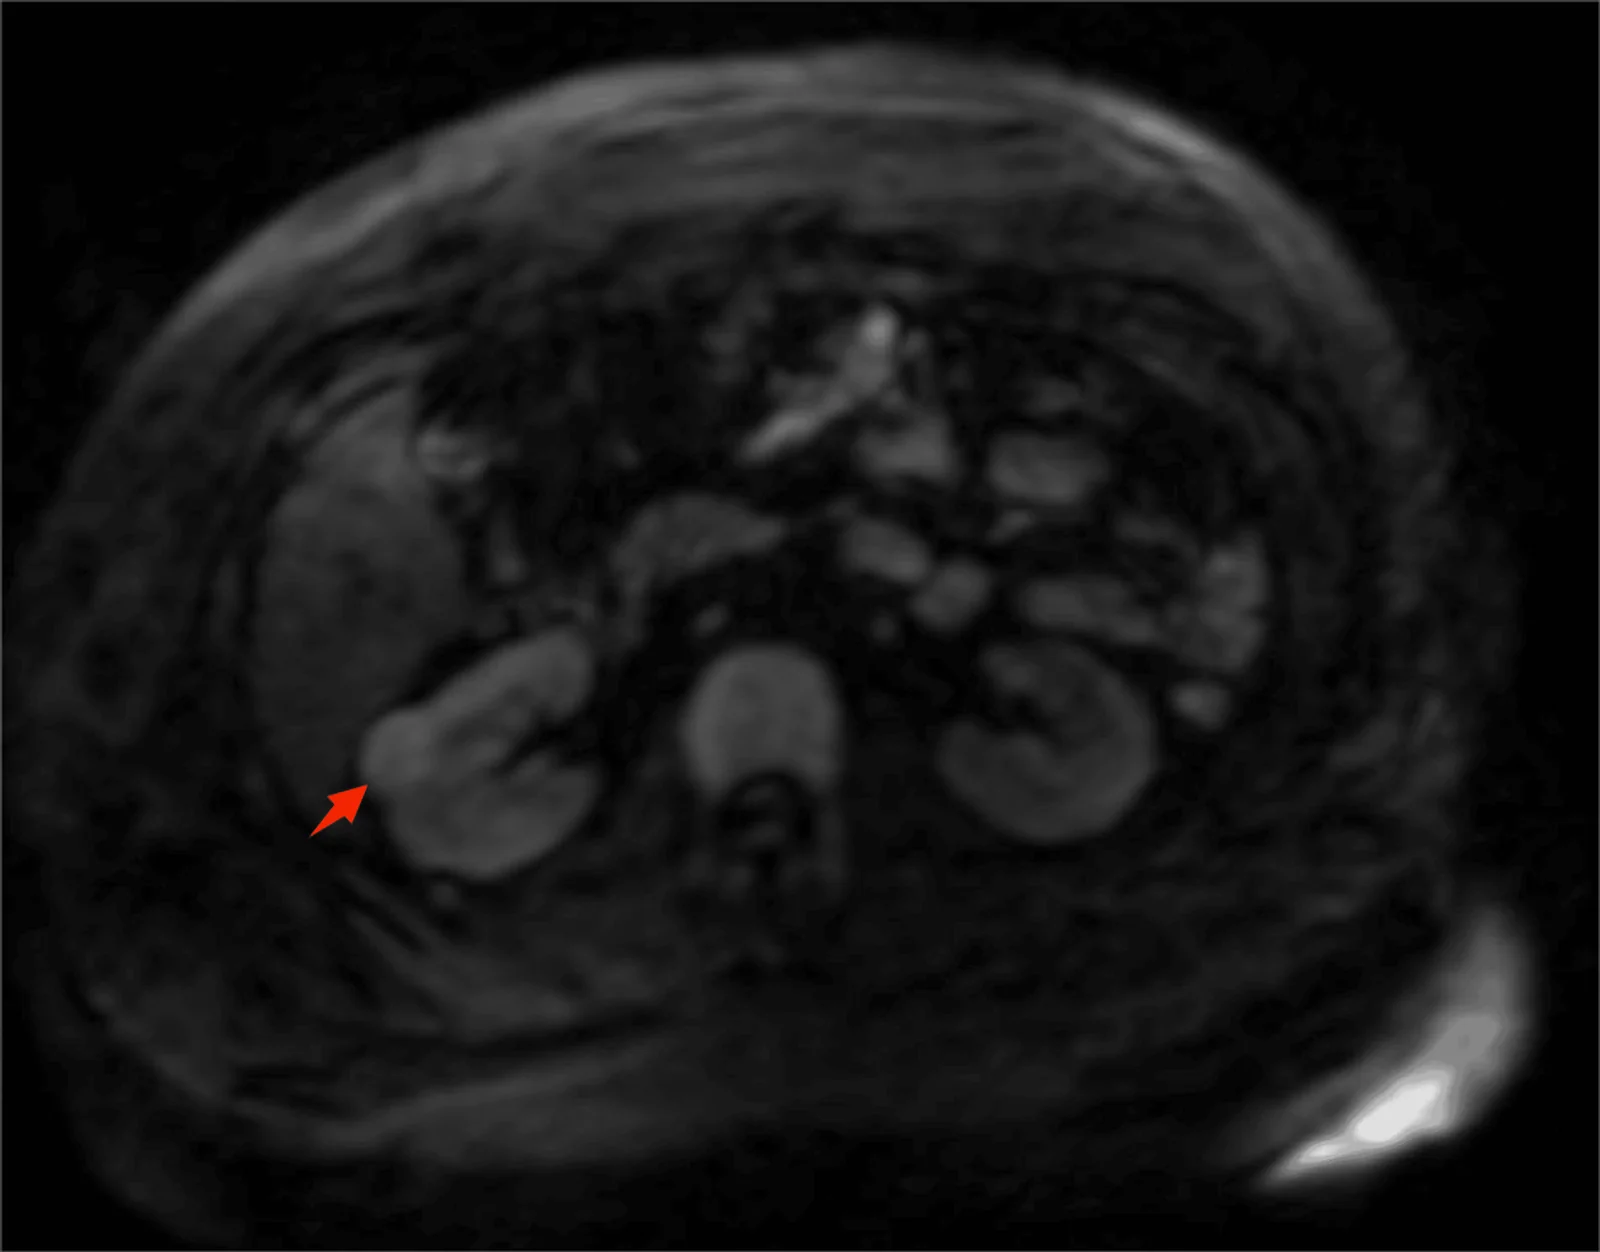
DWI showing high signal intensity (arrow) DWI: diffusion-weighted imaging
